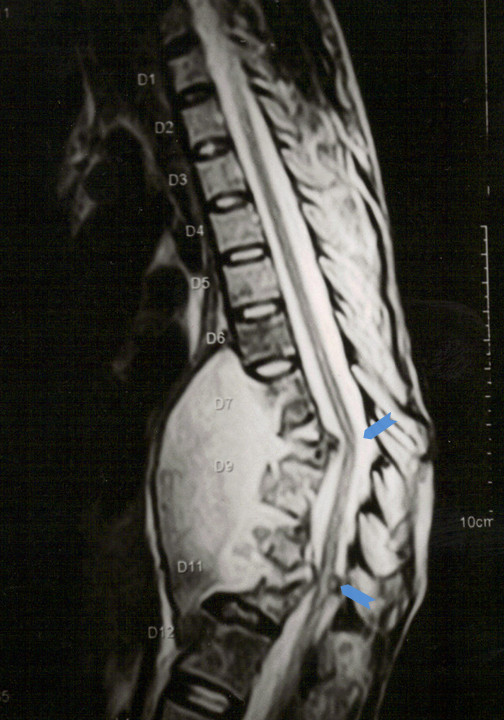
Sagittal MR image showing a huge prevertebral tubercular abscess extending from D6 to D12 levels with severe destruction vertebral bodies of D7 to D12 resulting in anterior angulation of spinal column leading to kinking of spinal cord at D7/8 and D10/11 with signal changes (arrowheads).
